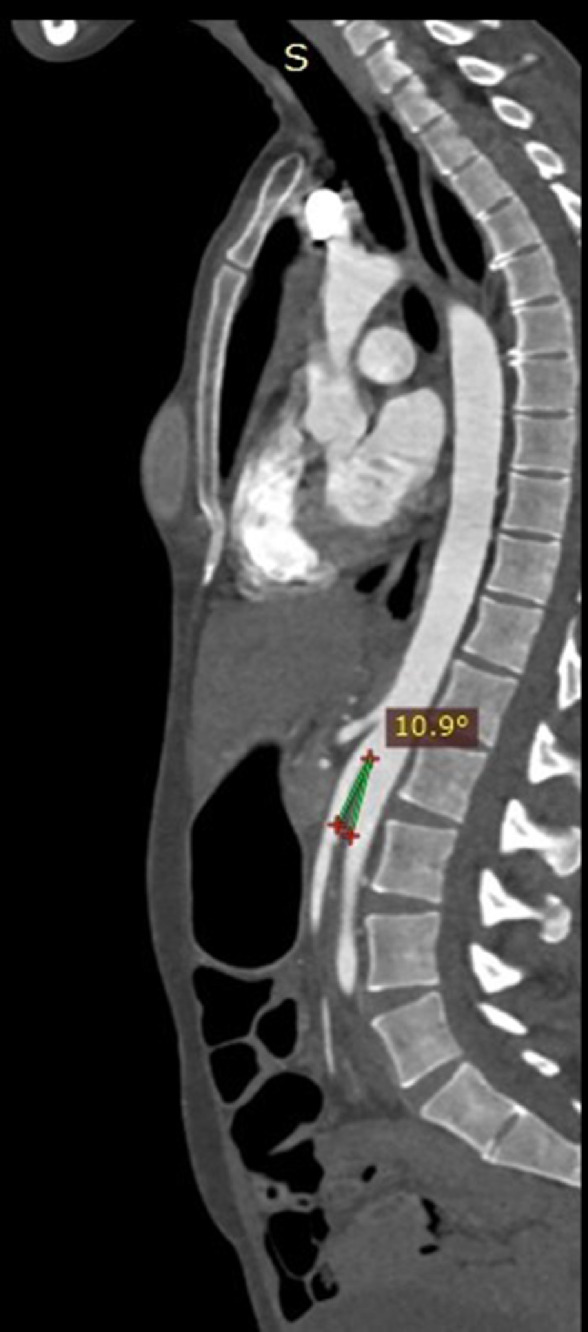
This figure shows the angiographic phase on the enhanced CT with the reduced angle between the abdominal aorta and the superior mesenteric artery.
